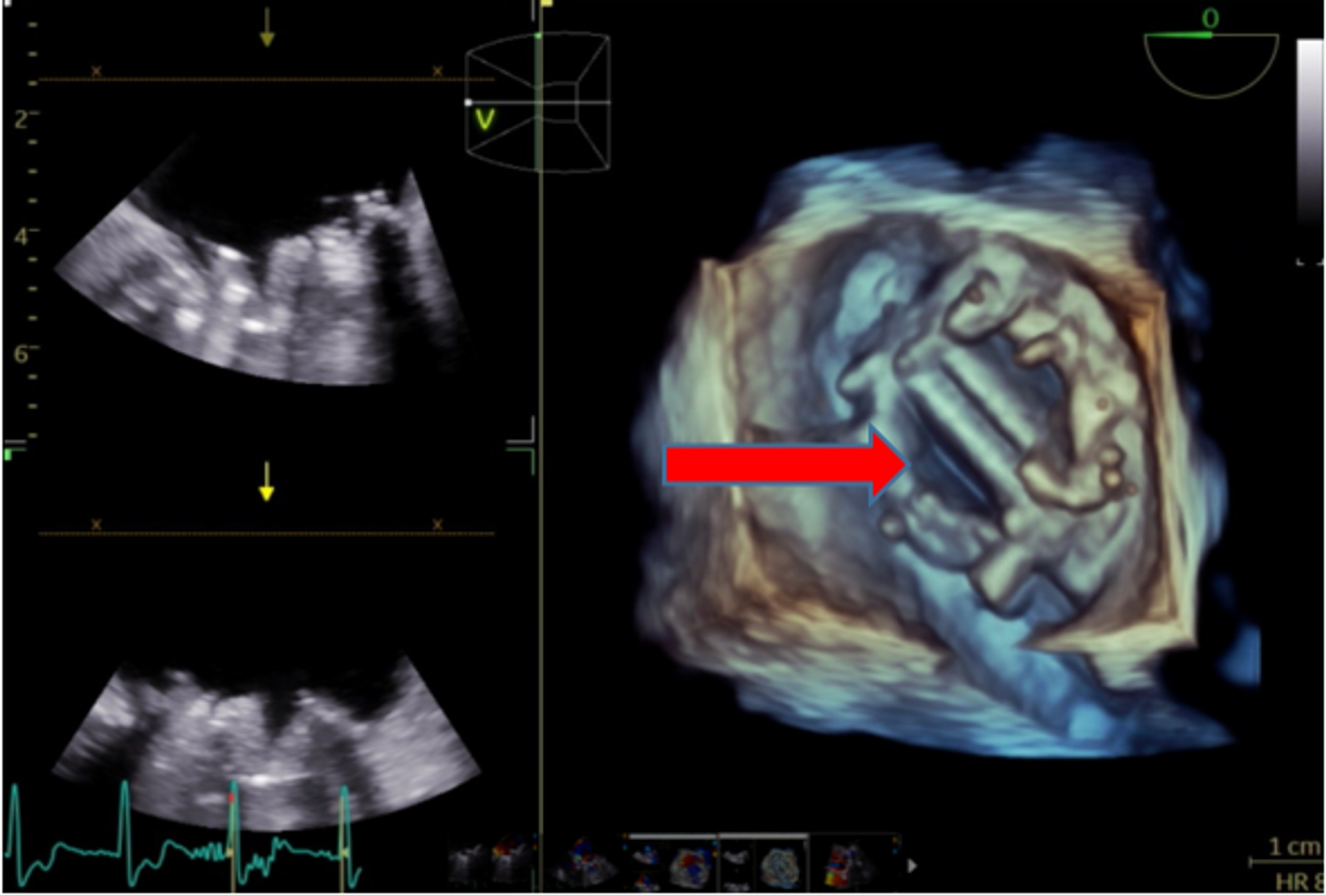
3D trans-esophageal echocardiogram of mitral valve and prosthesis (red arrow)
